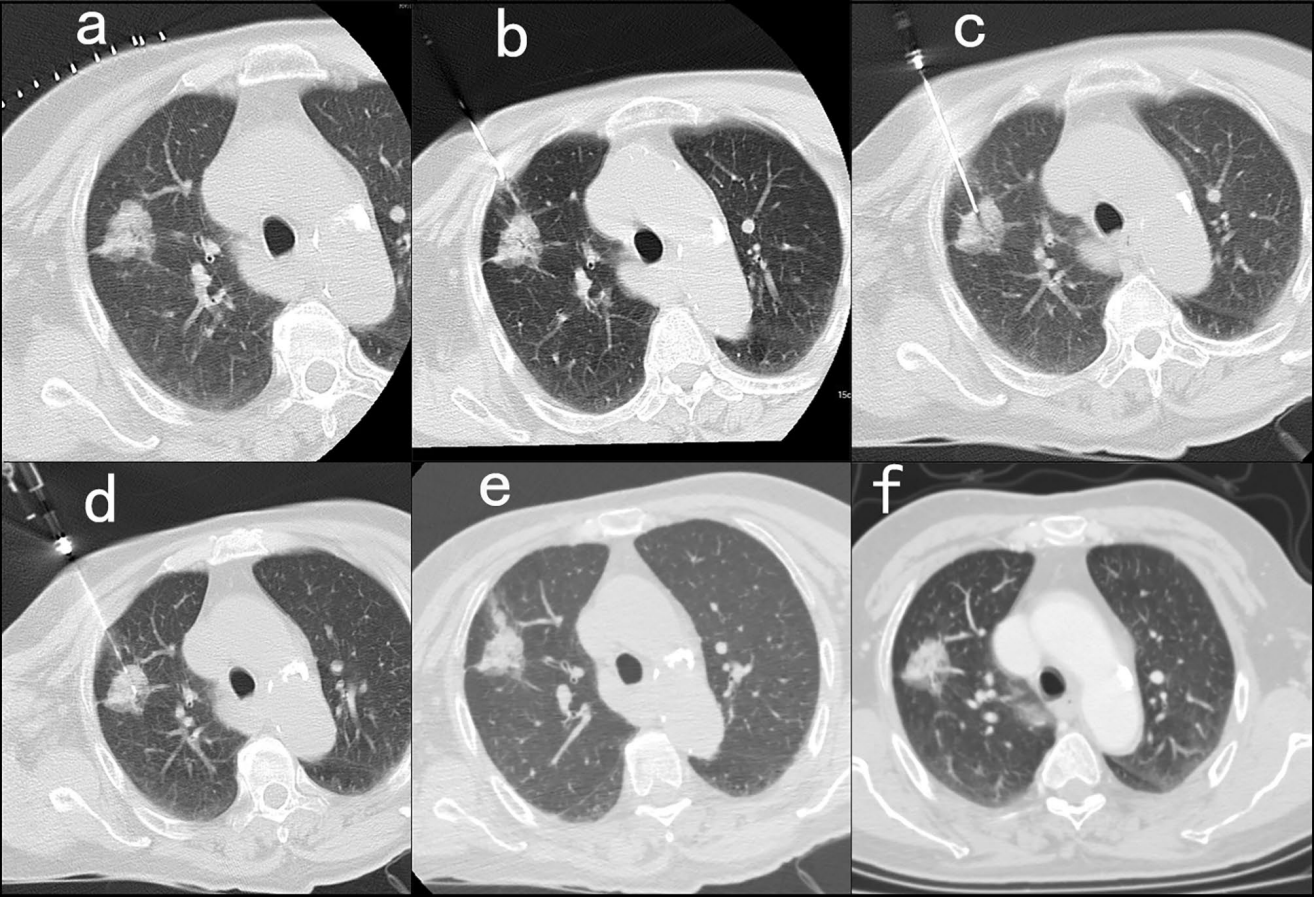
The process of plugging the puncture channel of a 64-year-old female patient: (a) The patient lies on the CT scan bed on her back, and a fence-like locator is placed on the skin surface. (b) The coaxial needle is punctured to the outside of the pleura. (c) After adjusting the direction, the coaxial needle reaches the edge of the nodule of the right upper lobe. (d) An 18G biopsy needle is inserted to cut the tissue. (e) When the coaxial needle is withdrawn, the plugging material is injected into the pleura and lung parenchyma, and the gelatin sponge slurry forms a striped shadow indicating high density. (f) The gelatin sponge slurry disappeared after 31 days, and the pathology report is lung adenocarcinoma.
